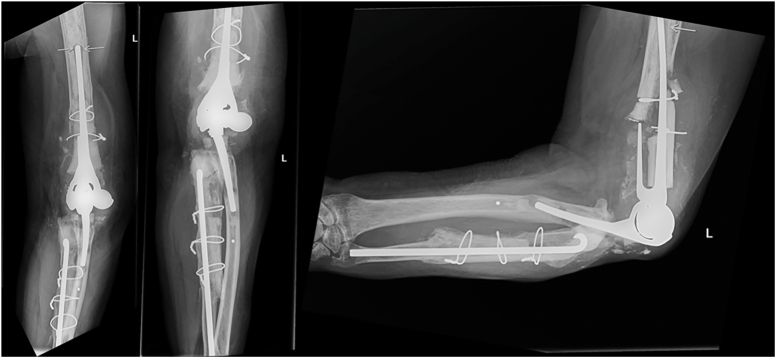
Radiographs from 2020 showing progressive humeral component loosening, with widening radiolucent lines along the proximal stem, loss of integrity of the distal humeral cerclage construct, and increasing apex-anterior angulation of the distal humeral segment. The proximal cerclage wire has eroded into the anterior cortex. The biologically reconstructed ulna remains structurally intact, though new radiolucent lines are visible around the radially implanted ulnar component.
